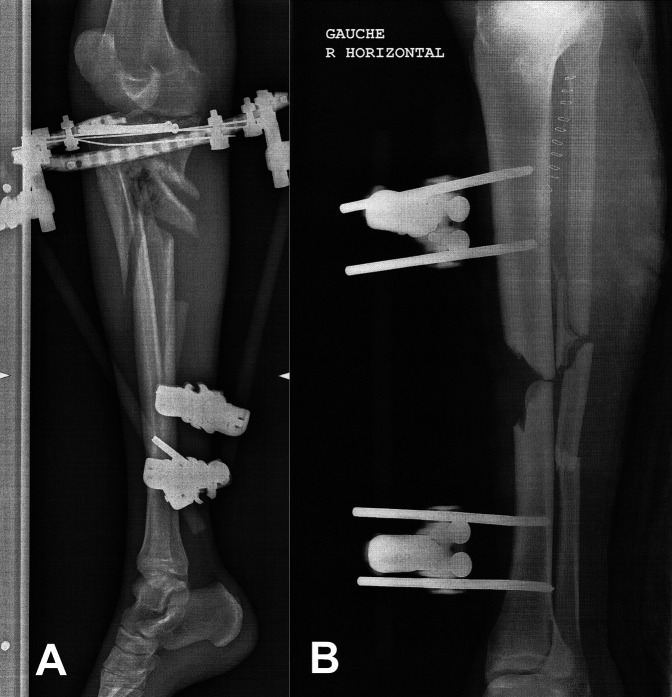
Radiograph after the first surgery for the management in emergency of the road accident: A, right side and (B) left side
